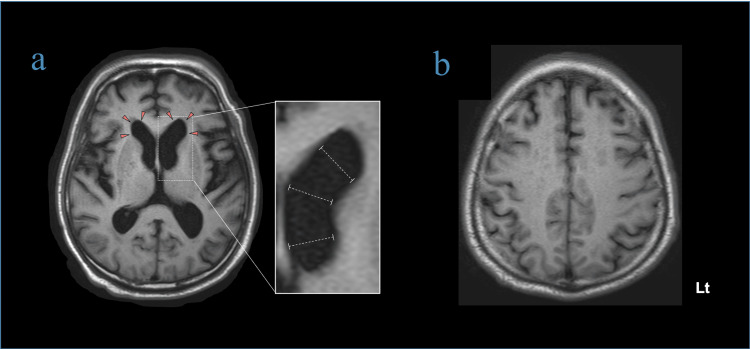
Axial T1-weighted brain MRI a. Mild ventricular enlargement is observed bilaterally at the anterior horn (red arrowheads). Diameters at the distal, mid, and proximal portions are approximately equal, forming a pattern termed the “boiled sausage sign.” b. Cortical atrophy is not observed. Narrowing of the cerebral sulci in the high convexity region is not observed.
